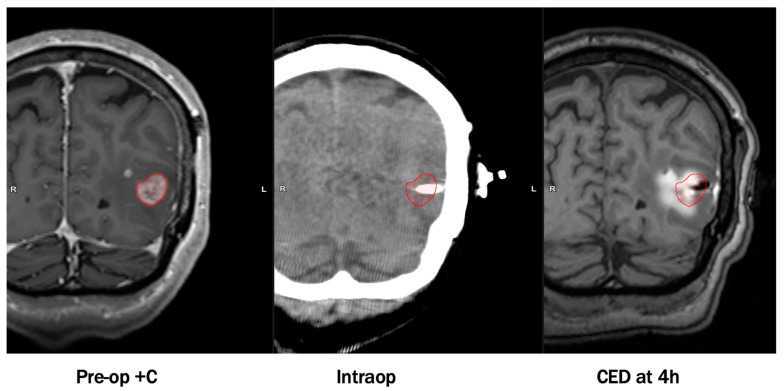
An example of an infusion performed by convection-enhanced delivery (CED) visualized on magnetic resonance imaging (MRI) with co-convected contrast. Images were obtained with permission from Dr. Michael Vogelbaum and Moffitt Cancer Center.
